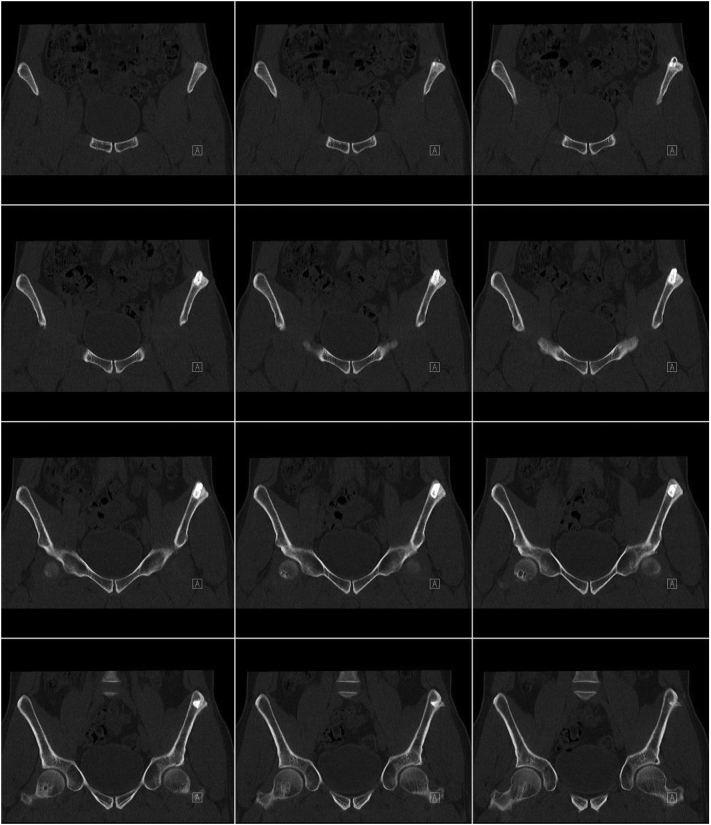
Series of coronal reconstruction CT images of the pelvis 12 months after defect filling at the left iliac crest with a 15 × 15 × 10 mm scaffold block from anterior to posterior. The series shows complete bony integration of the biomaterial into the surrounding host bone with bridging of the bone-implant interface with newly formed bone.
